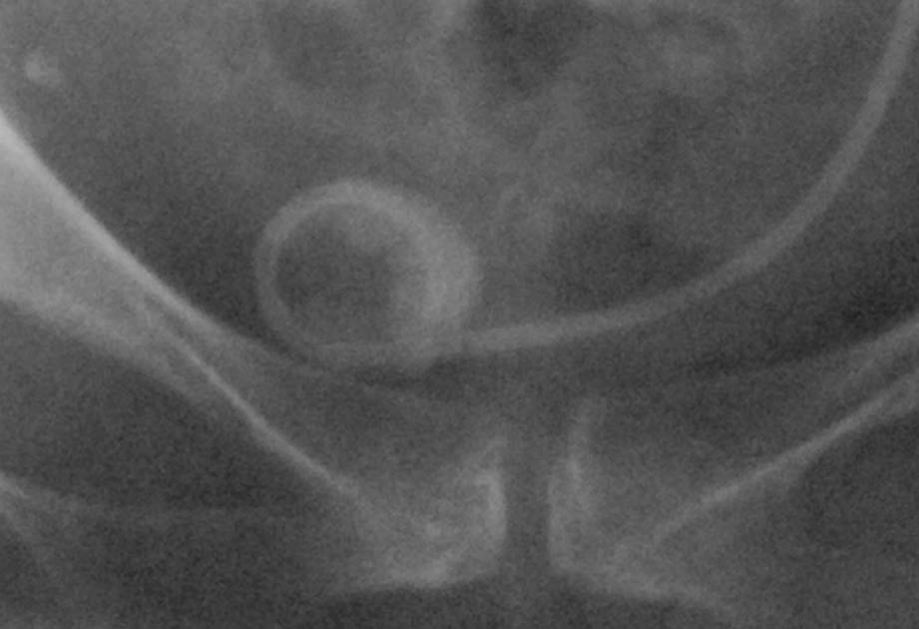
Stent encrustration of bladder coil (radiography aspect).
